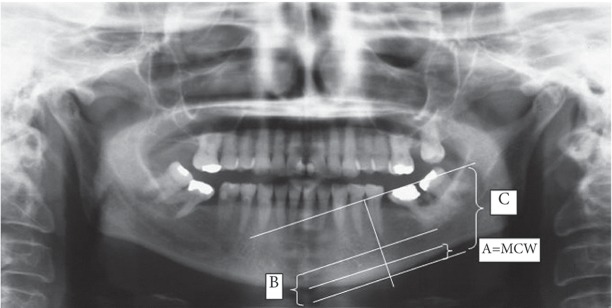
Panoramic radiography. Measurements of the quantitative indices. For more details refer to the text (MCW=A, PMI= A/B, M/M ratio=C/B).
